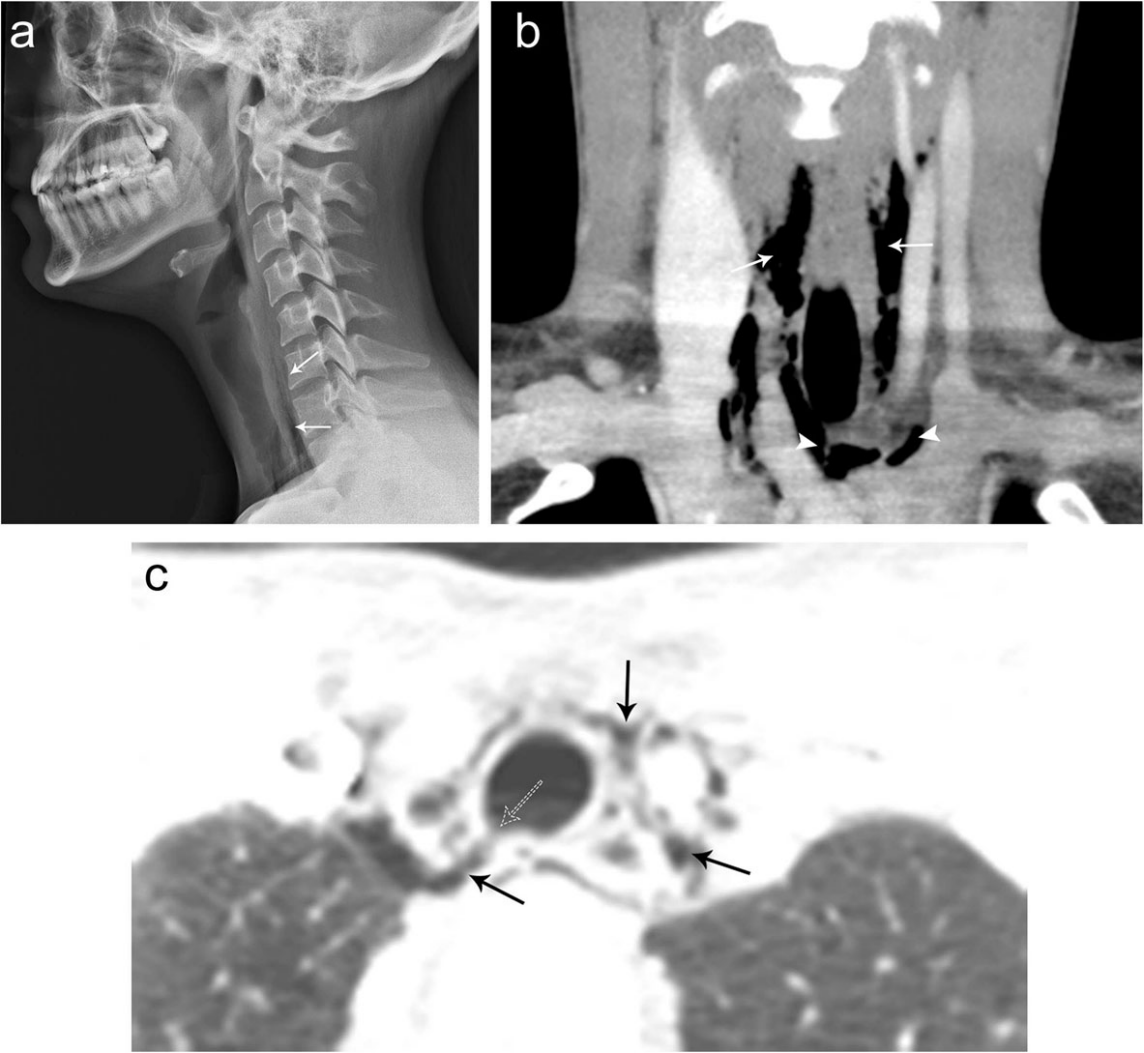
Subcutaneous emphysema and pneumomediastinum. Lateral cervical spine radiograph (a) showing linear streaks of air (arrows) tracking along the prevertebral soft tissues of the thoracic inlet consistent with subcutaneous emphysema. Coronal (b) contrast CT image demonstrates subcutaneous air (arrows) tracking along the cervical soft tissues and fascial planes extending into the superior mediastinum (arrowhead). Axial CT chest image in lung (c) windows demonstrating air (solid arrows) within fascial planes of the superior mediastinum. Note the tracheal defect (dashed arrow) which was the source of the pneumomediastinum and subcutaneous emphysema on the lung window images
